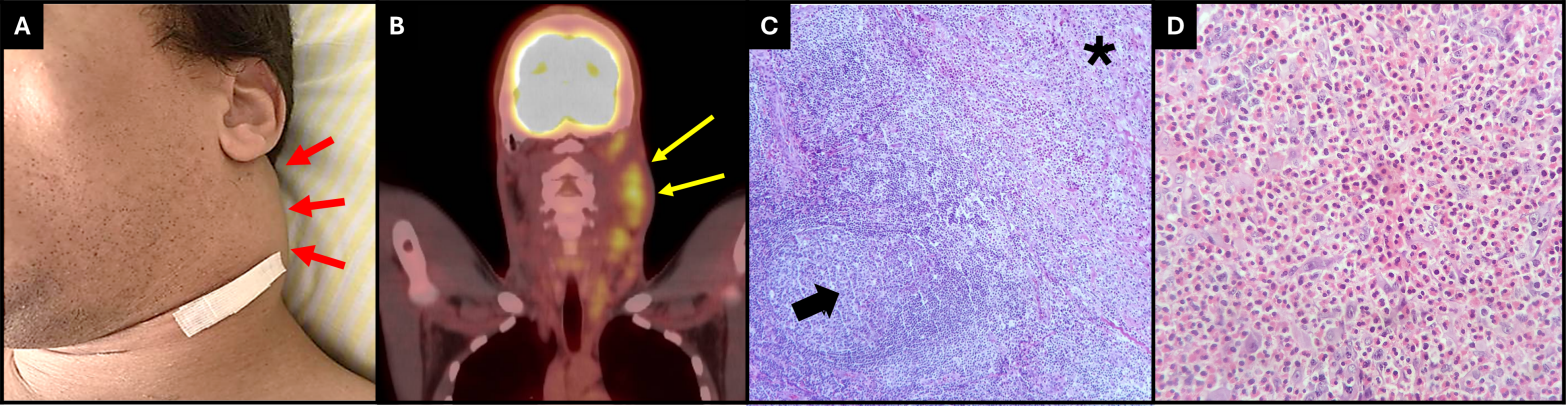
(A) Picture revealing the patient’s left neck mass (red arrows) (B) PET-CT scan reveals a high uptake of tracer in left cervical lymphonodes. (yellow arrows). (C) Lymph node showing zone of eosinophil-rich polimormorphic infiltrate, proliferation of reactive endothelium, and interstitial collagen deposition in a nodular fashion (black asterisk). The rest of the nodal parenchyma reveals numerous reactive lymphoid follicles (black arrow) (HE, 100×). (D) High magnification of the eosinophil-rich polymorphic infiltrate (HE, 400×).
